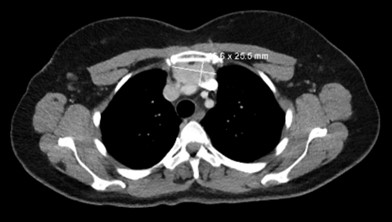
Thoracic CT scan demonstrating a thymic mass.
